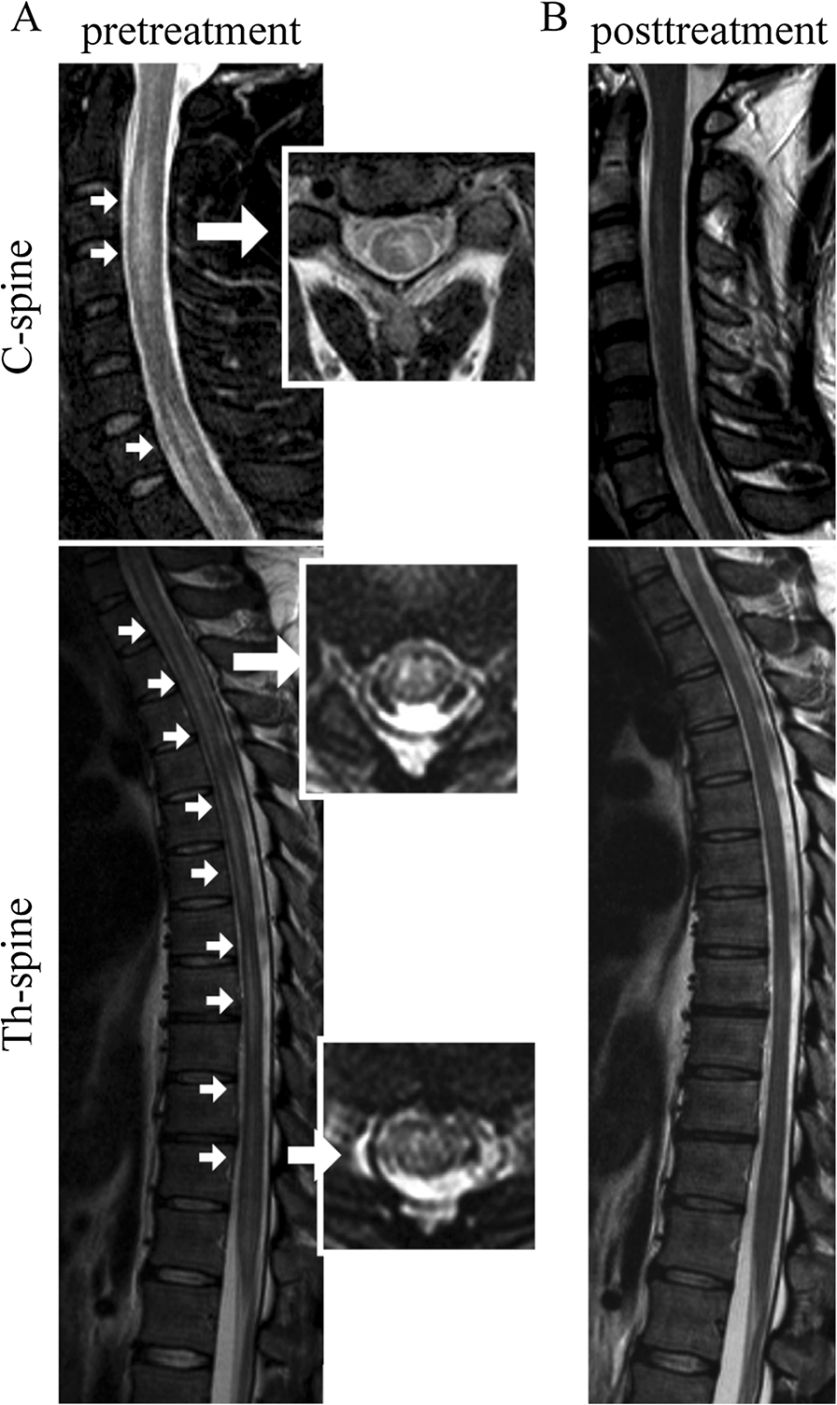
T2-imaging MRI of cervical (C)/thoracic (Th) spinal cord on admission (A) and after treatment (B). On admission (day 10), there was a longitudinal T2 high intensity area, extending from C2 to the conus (arrowheads), but the lesion resolved completely after treatment (day 20).
